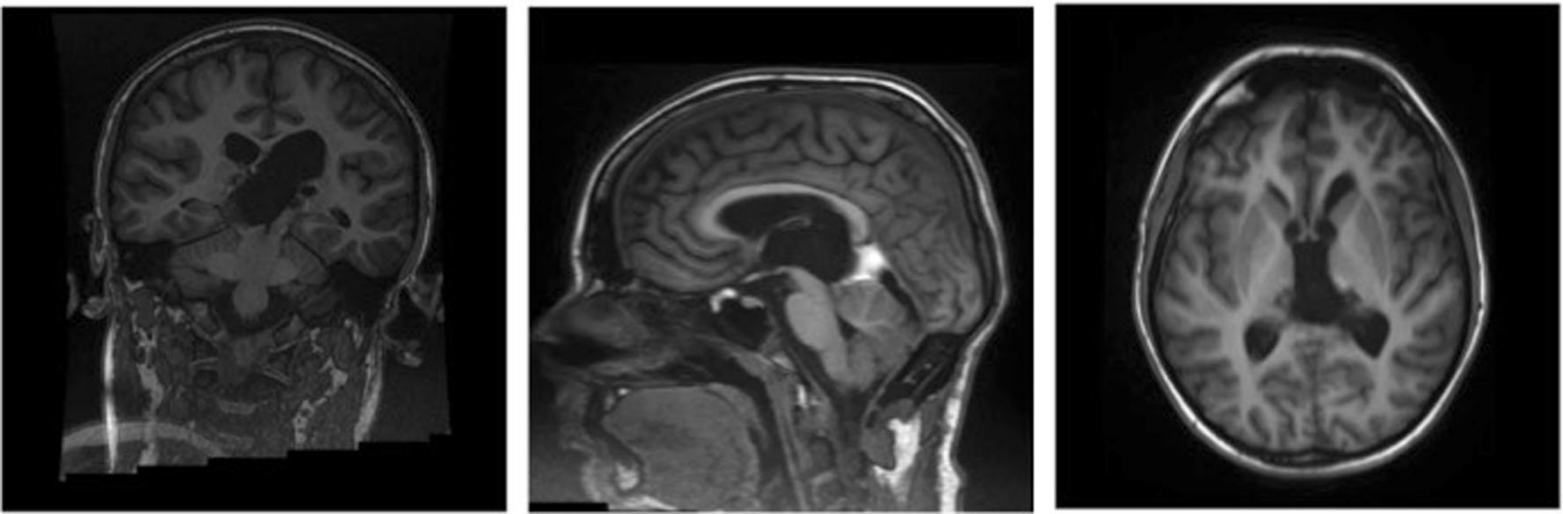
Postoperative MRI images showing complete resection of tumour at the end of procedure
